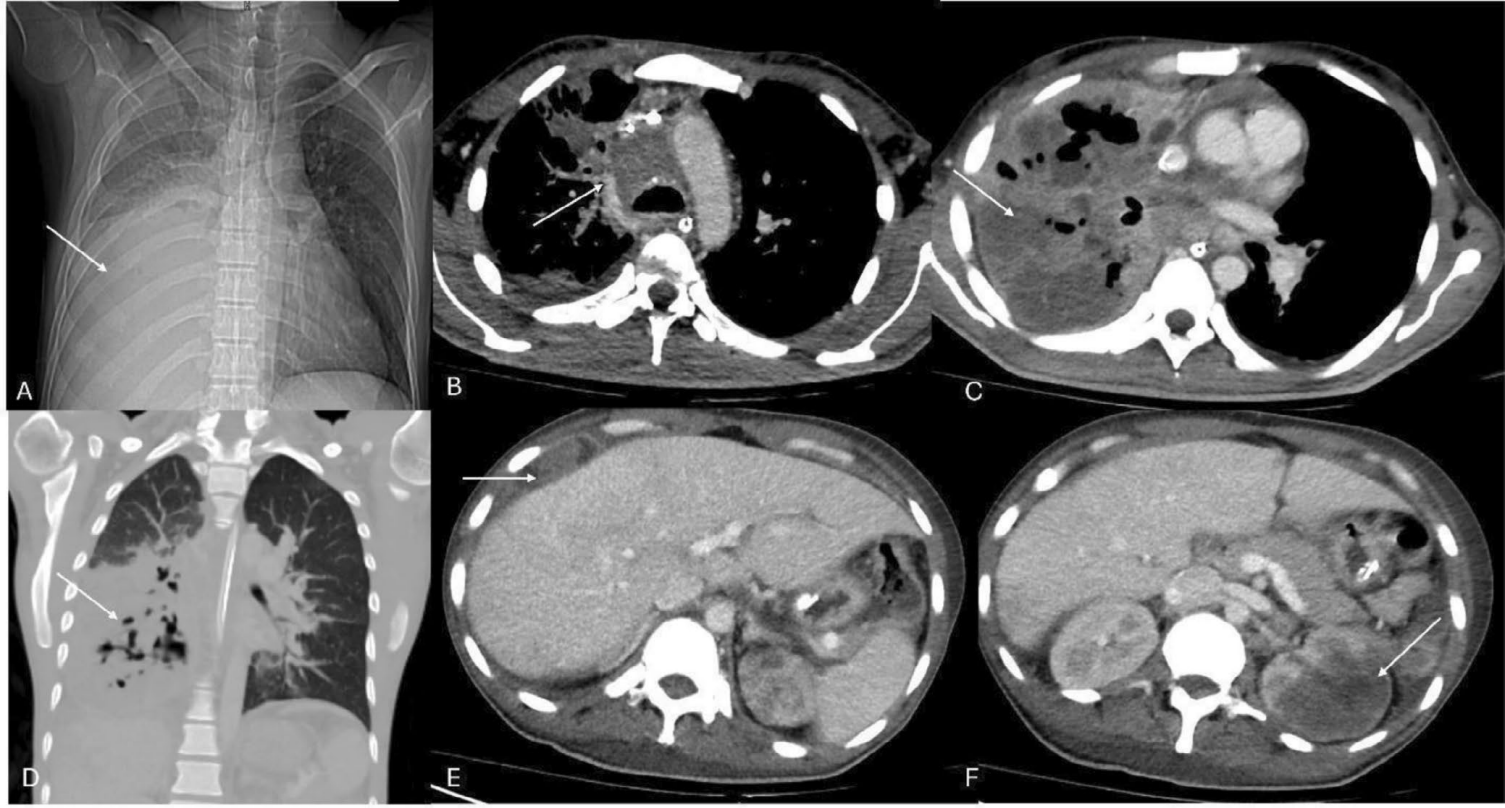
(A) Chest x‐ray showing large consolidative opacity in the right lower lung zone (arrow). (B, C) Axial CT chest image showing multifocal low attenuation infiltrative masses in the mediastinum and right lung (arrows). (D) Areas of cavitation and necrosis demonstrated on coronal CT image in lung window settings (arrow). (E, F) Axial CT images from the upper abdomen showed perihepatic lesions (arrow in E) and left renal lesions (arrow in F).
